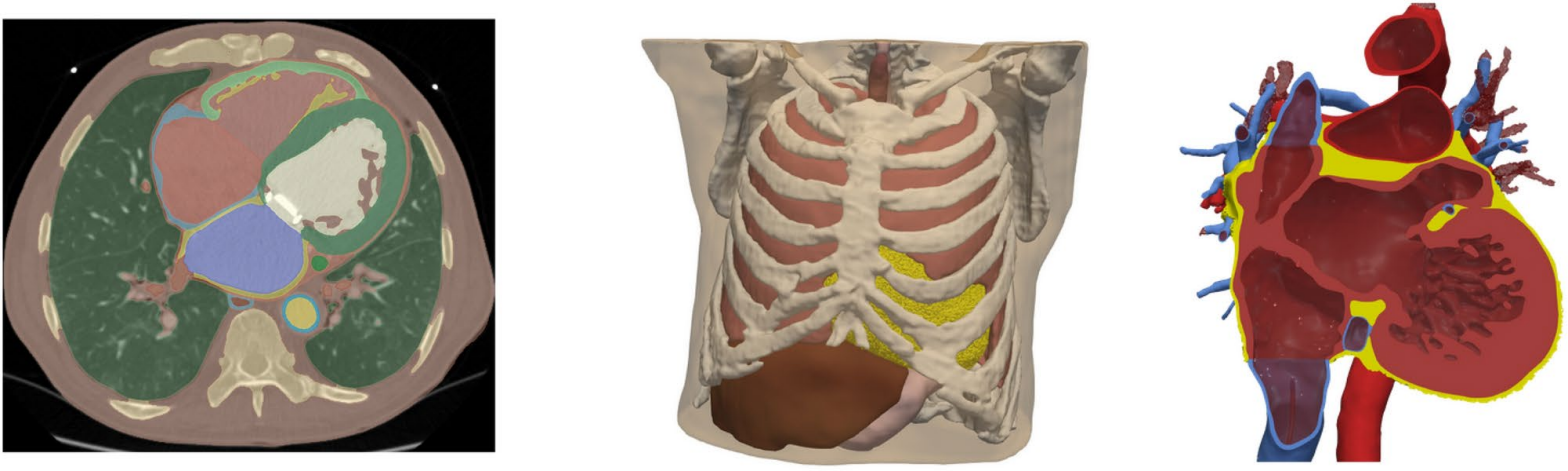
Left: The CT scan image with our multi-label segmentation, consisting of a total of 35 distinct labels identifying each anatomical structure. Middle: The discretized torso geometry with all organs, using tetrahedral elements. Right: The detailed cardiac geometry with trabeculae, pericardium, blood vessels and their walls.
